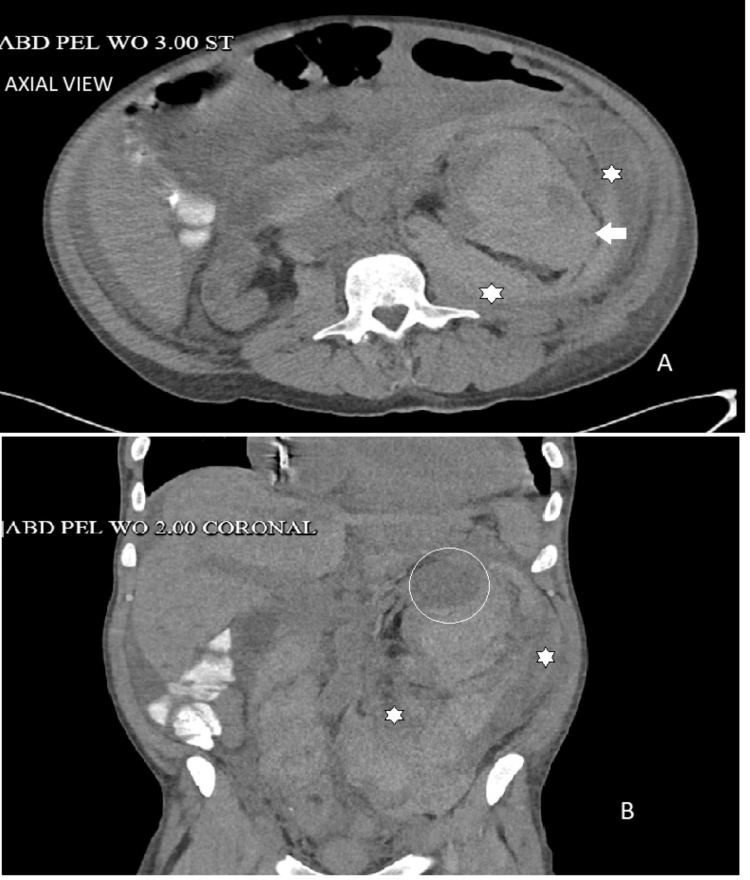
Computed tomography angiography showing the left kidney (white arrow) with extensive perinephric and retroperitoneal blood (asterisk marks in image A, axial view, and image B, coronal view), with a renal cyst (white circle) in image B
